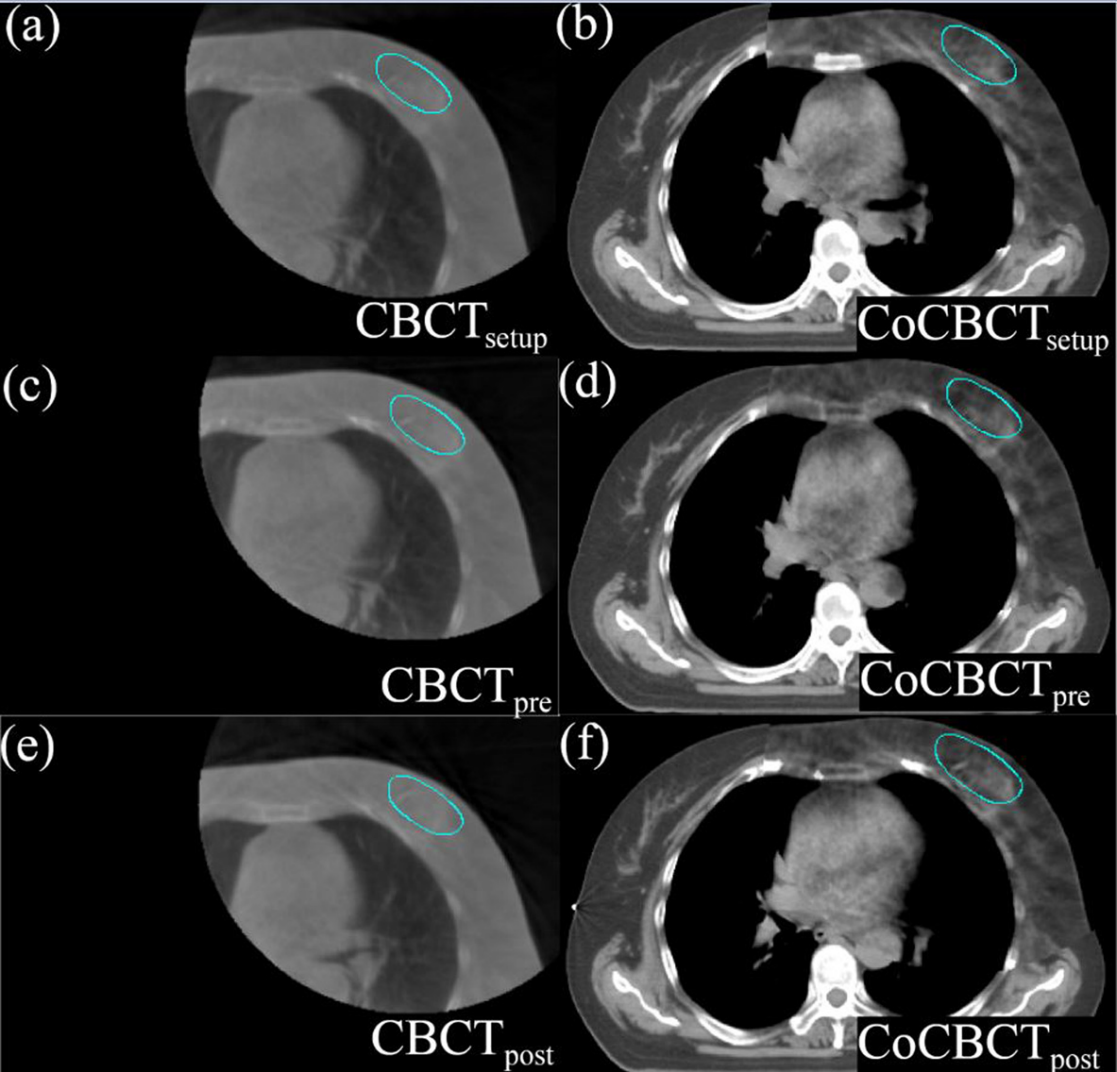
The axial views of original CBCT images and corrected CBCT images for one fraction of patient2. (a) CBCT after skin-marker setup (b) corrected CBCT after skin-marker setup (c) CBCT after online setup correction (d) corrected CBCT after online setup correction (e) CBCT after treatment delivery (f) corrected CBCT after treatment delivery.
